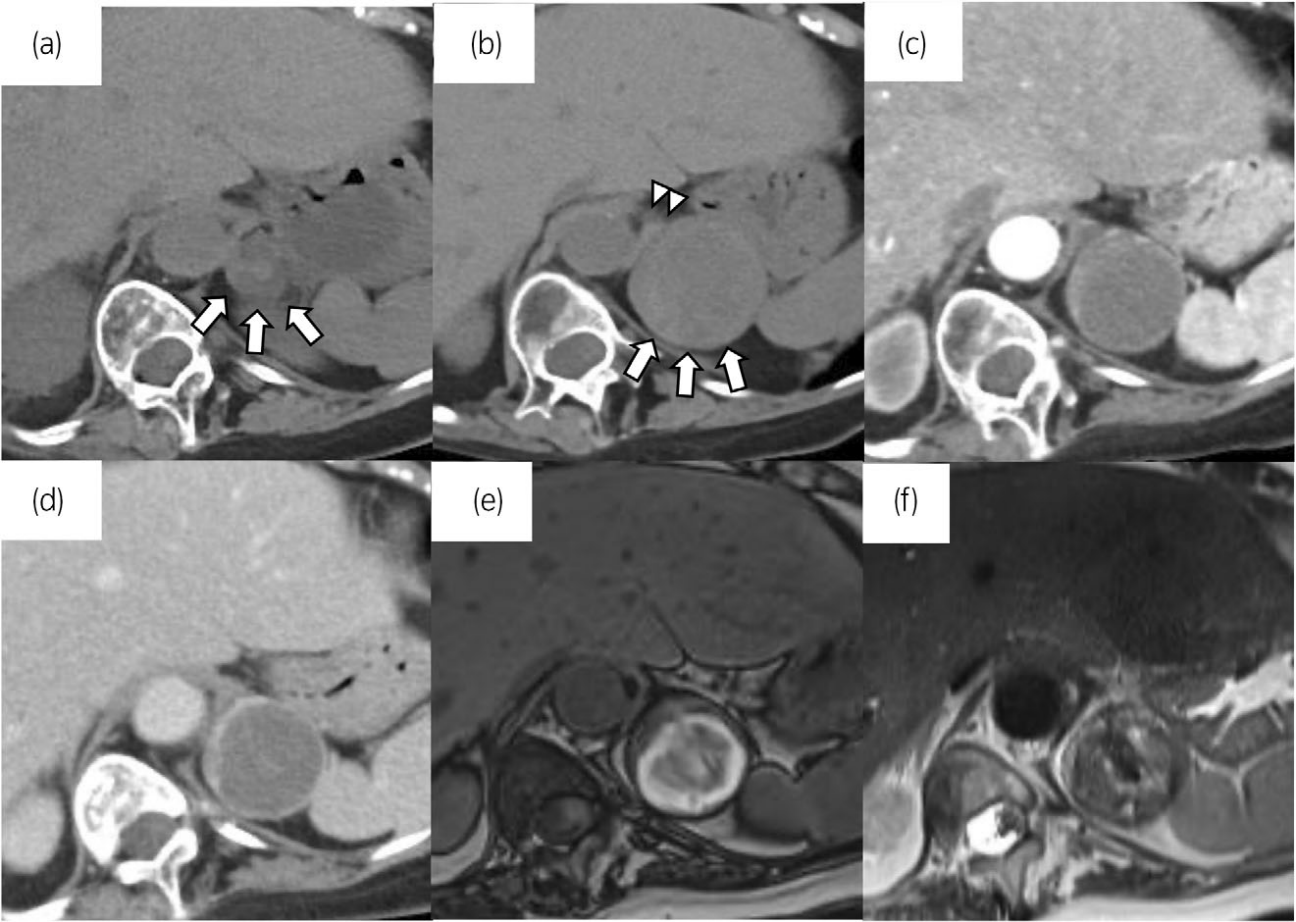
Findings of CT and MRI. (a) Plain CT 1 year before surgery. A CT scan showed a 23‐mm left adrenal tumor that was diagnosed as an adrenal cortical adenoma. White arrows indicate the adrenal tumor. (b) Plain CT. White arrowheads indicate the left adrenal gland. White arrows indicate the adrenal tumor. Calcification of the tumor was not observed. (c) Arterial phase on contrast‐enhanced CT. (d) Portal phase of contrast‐enhanced CT. The tumor margins are clearly contrasted, and the inside of the tumor is lightly contrasted. (e, f) On MRI, an area inside the tumor with high intensity on T1‐weighted images (e) and low intensity on T2‐weighted images (f) is observed, and intratumoral hemorrhage is suspected.
